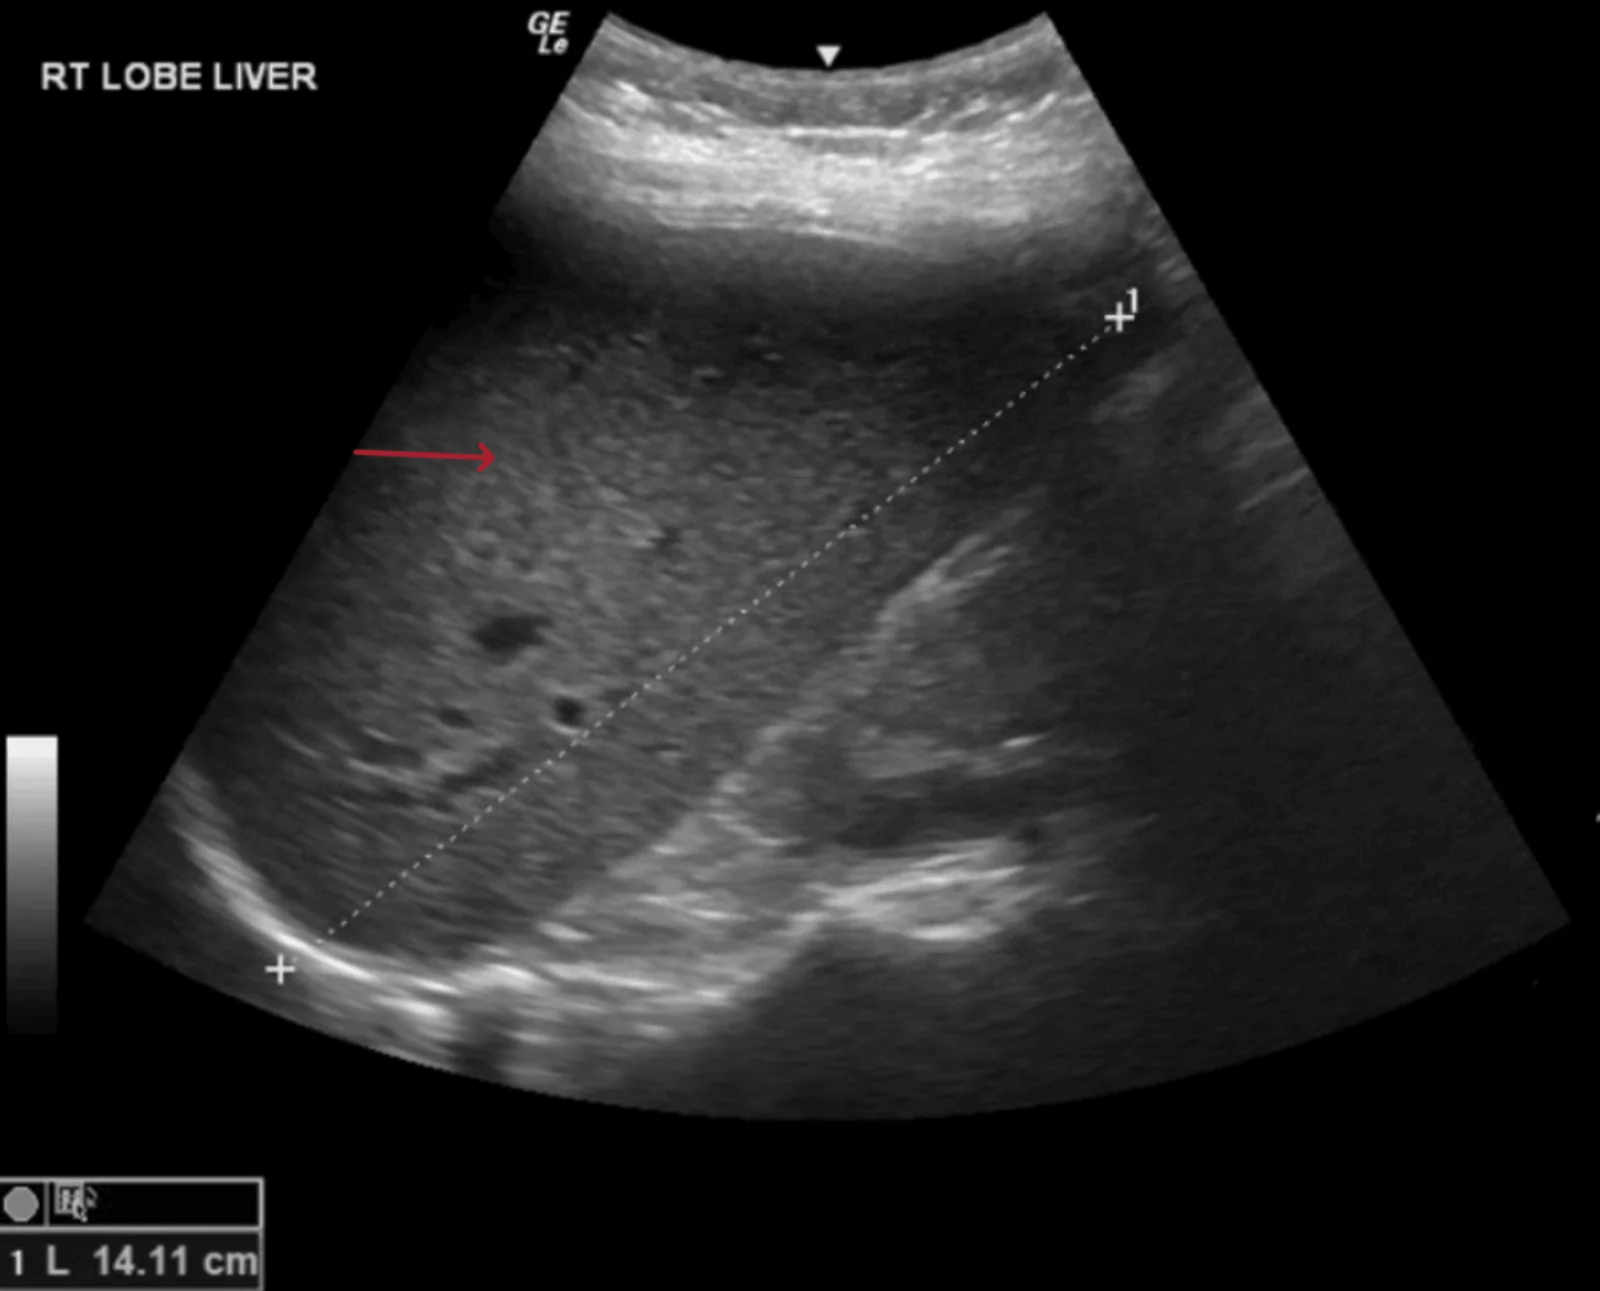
Hepatobiliary ultrasound demonstrating normal liver parenchyma (as indicated by the red arrow) and no biliary pathology. Ultrasound examination of the hepatobiliary system demonstrating normal liver size, smooth contour, and homogeneous echotexture without focal lesions. The portal vein is normal in caliber. The gallbladder shows no wall thickening, pericholecystic fluid, or intraluminal sludge. The common bile duct and intrahepatic biliary ducts are within normal limits. The pancreas is partially obscured by bowel gas but the visualized portions are unremarkable. No ascites or lymphadenopathy is identified.
